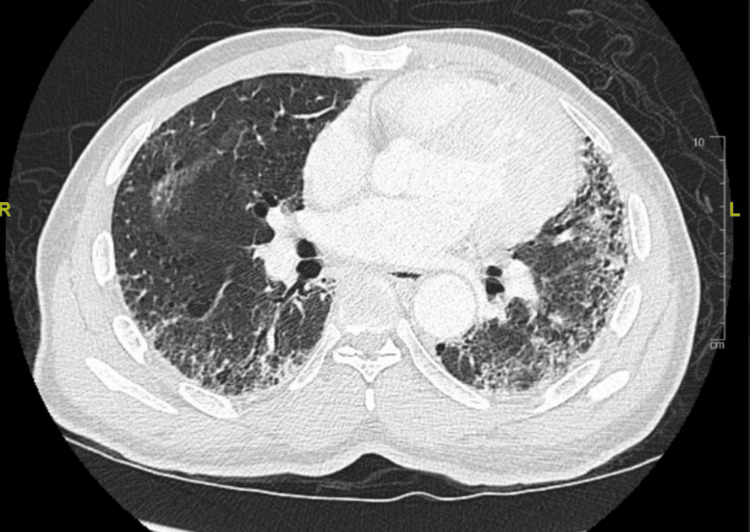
CT chest 3 months after chemotherapy showing changes suggesting pulmonary fibrosis
